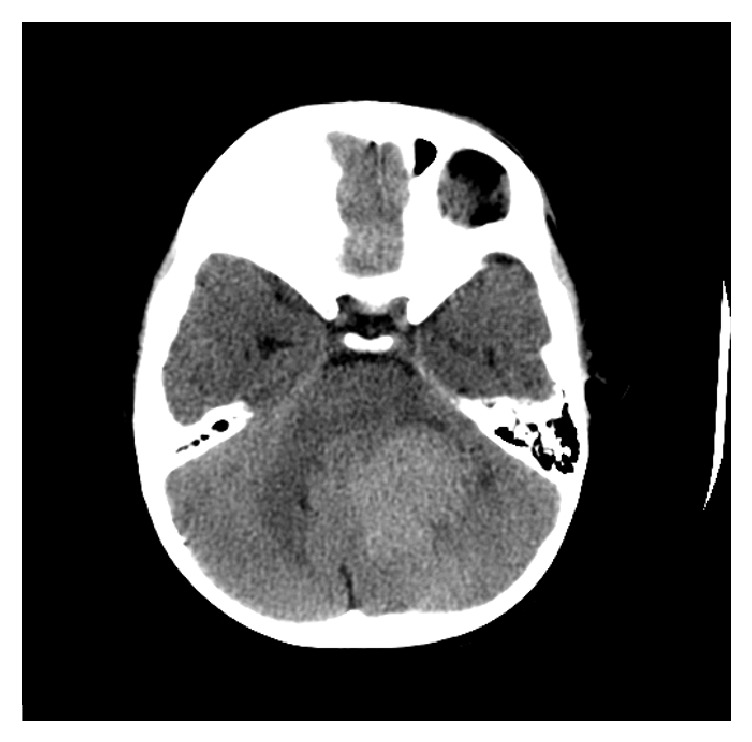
Axial CT of the brain revealed left-sided posterior fossa hyperdense mass compressing the fourth ventricle and brainstem.
